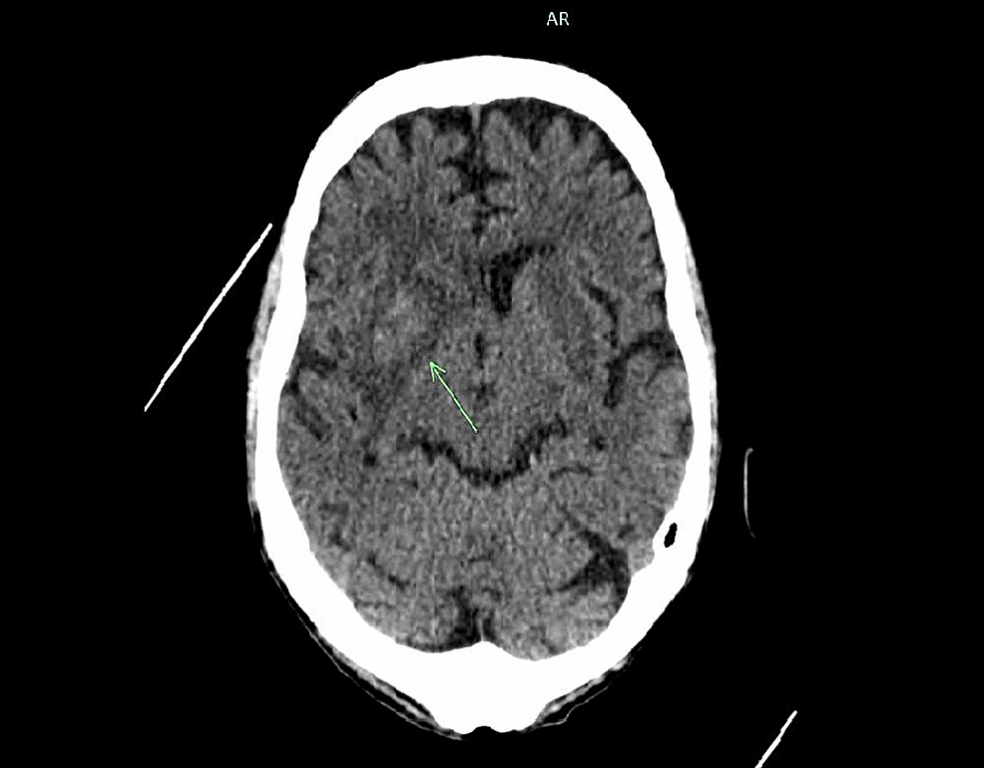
CT non-contrast The arrow indicates an intraparenchymal hemorrhage in the right basal ganglia.
